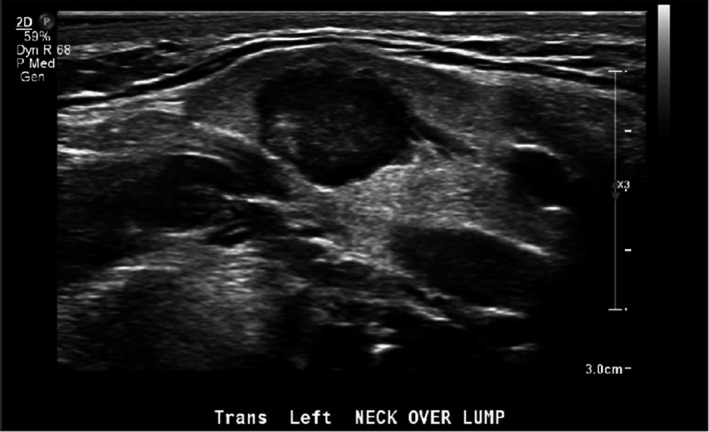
Ultrasound of the left submandibular gland showing a 1.6 × 1.2 × 1.3 centimeter irregularly marginated, hypovascular, and hypoechoic lesion
